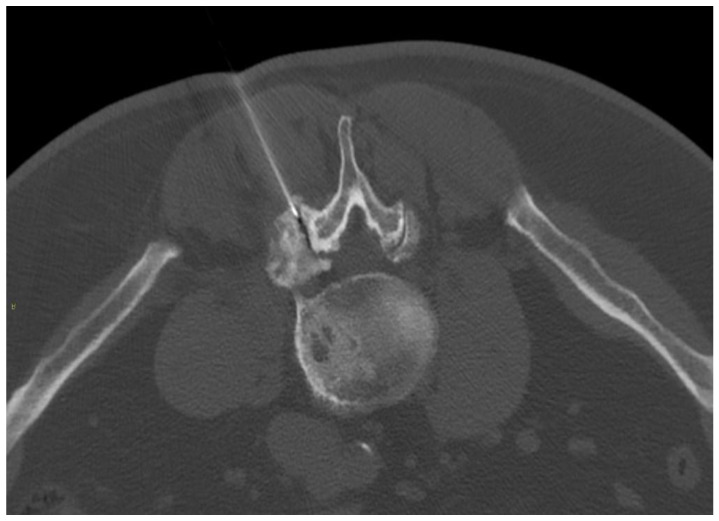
A 67-year-old male with severe lumbar facet joint arthritis underwent CT-guided L5-S1 right posterior joint corticosteroid injection.
